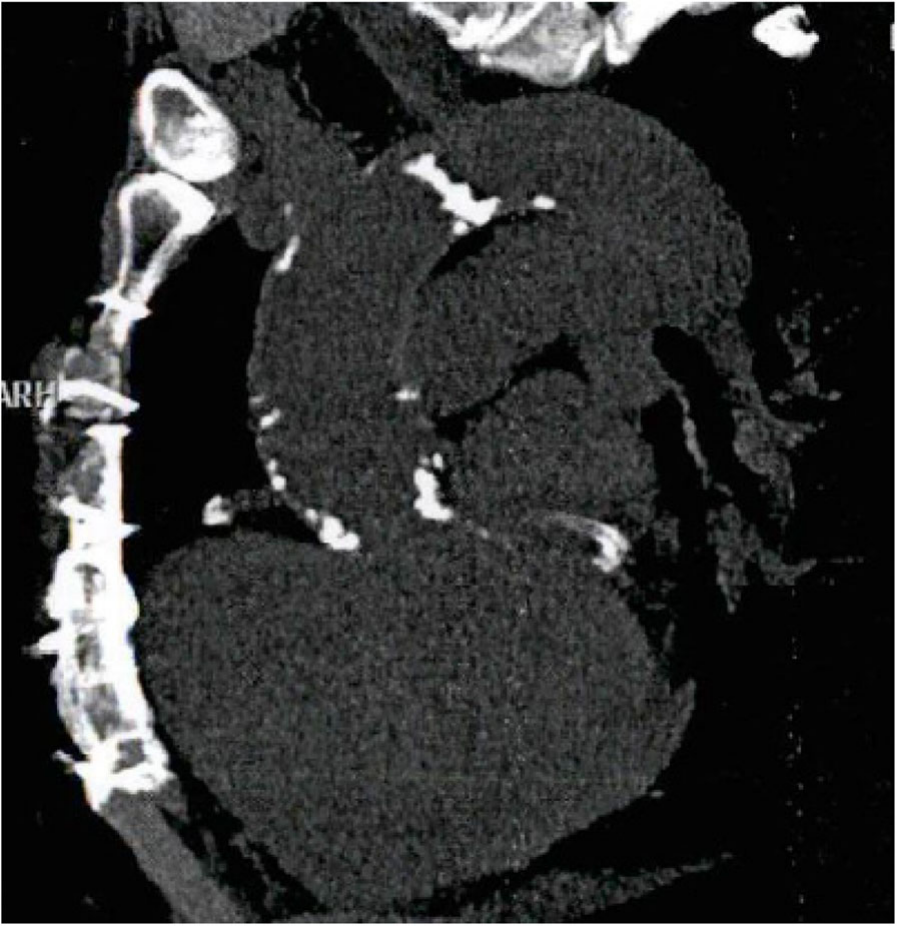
Preoperative computed tomography scan of the chest showing calcific root, ascending aorta and arch
